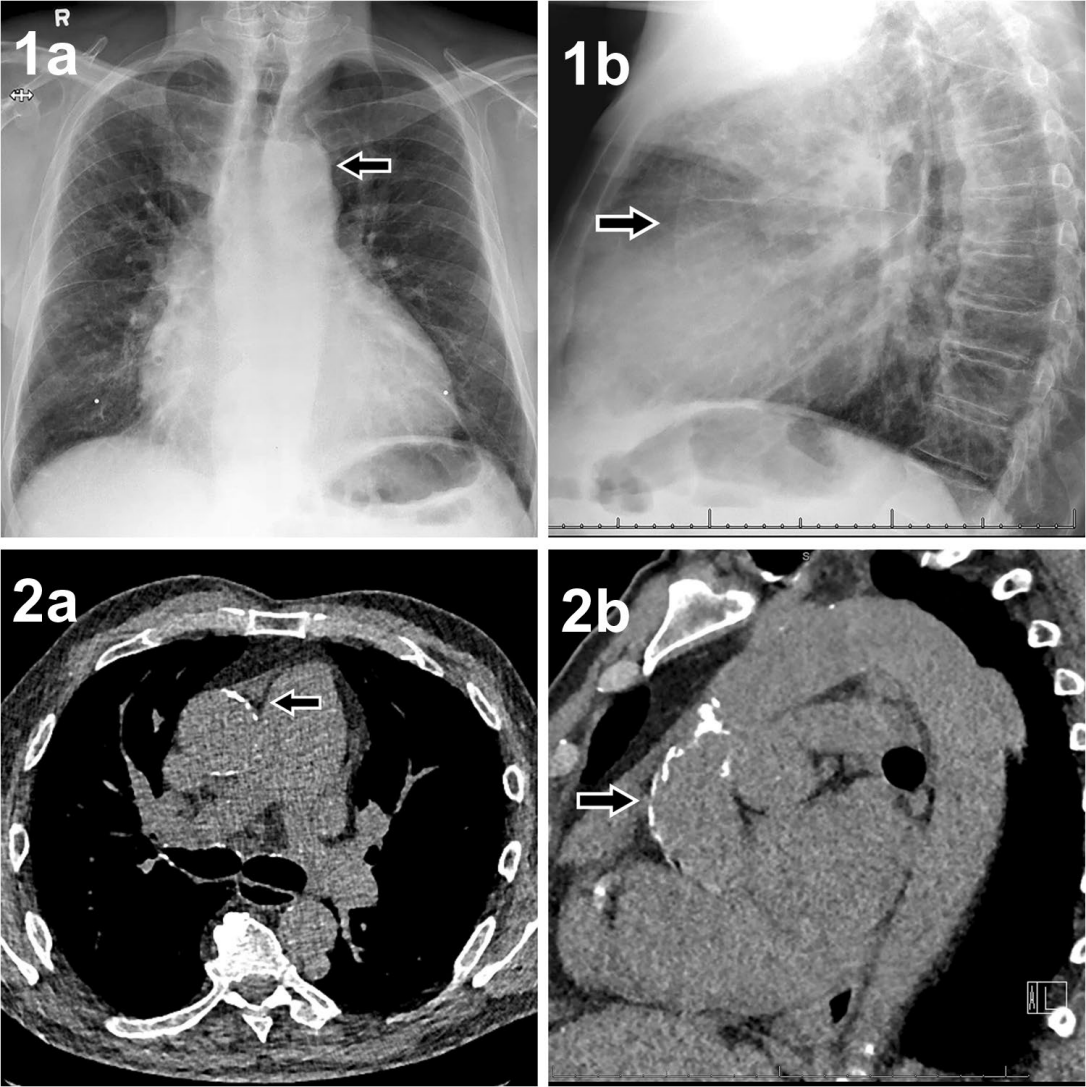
Sample images of CXR and noncontrast CT. Sample images of a 70-year-old male patient whose surgical approach was changed from surgical to transcatheter aortic valve replacement. 1: The posterior-anterior (1a) and lateral (1b) views of the preoperative CXR 2: Ascending aortic calcifications on the axial (2a) and sagittal (2b) plane of the noncontrast CT. The arrow in panel 1a indicates the aortic knob, where only modest calcification is seen. The arrow in panel 1b point at the ventral boundary of the aorta, where no clear calcifications seem to be present. The arrows in panels 2a and 2b indicate the extensive ventral calcifications, hampering aortic manipulation in this area
